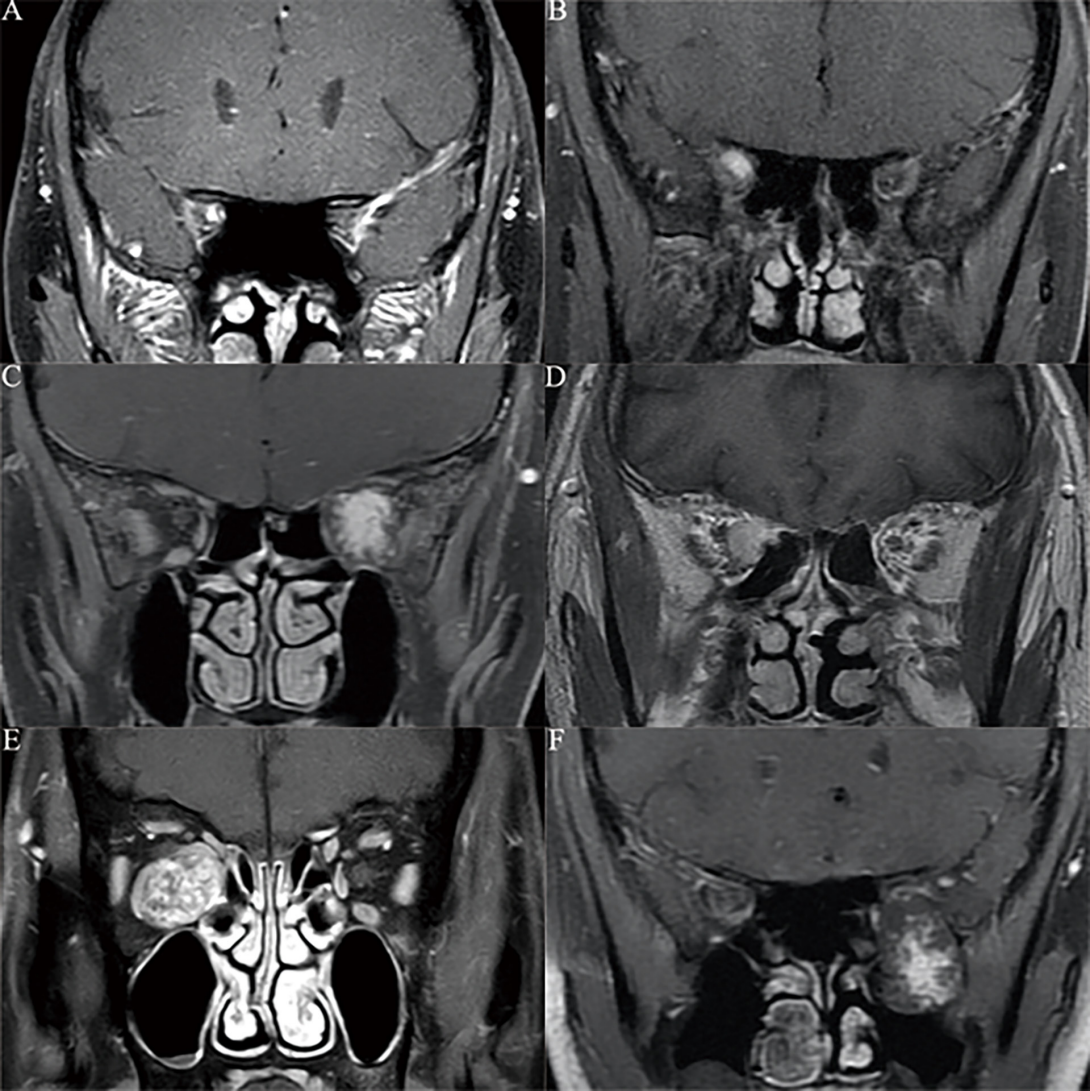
According to optic nerve MRI and intraoperative confirmation, we found the common location classification of orbital cavernous hemangioma. (A) MRI of optic nerve showed the tumor is located under the optic nerve sheath in the orbital apex area. (B) MRI of optic nerve showed the tumor is located in the common tendon ring of the orbital apex region. (C) MRI of optic nerve showed the tumor is located inside the muscle cone of the orbit. (D) MRI of optic nerve showed the tumor is located outside the muscle cone of the orbit. (E) MRI of optic nerve showed the tumor involved both the inside and outside communication region of the muscle cone. (F) MRI of optic nerve showed the tumor involved both the inside and outside communication region of the orbit. On all contrast-enhanced MRI images, we can see that cavernous hemangioma varies in size according to its location in the orbit, showing homogeneous or patchy enhancement.
